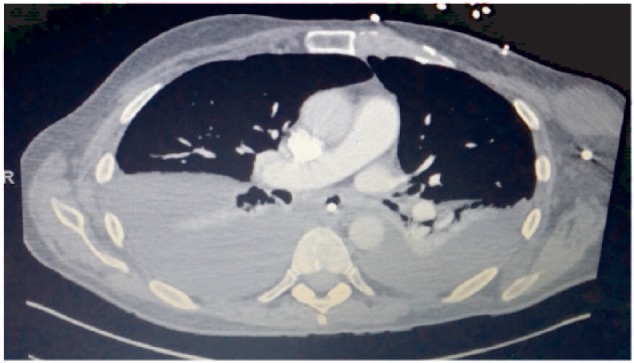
Computed tomography scan 1 week after the surgery—large right and moderate left pleural effusions.
